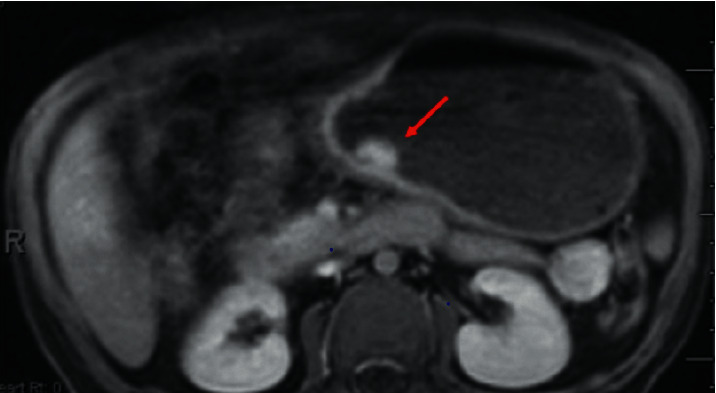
MRI of the abdomen and pelvis with and without contrast of patient demonstrating evidence, a 0.6 × 1 cm focal polypoid-like mass lesion was observed near the junction of the distal body/antrum of the stomach.
